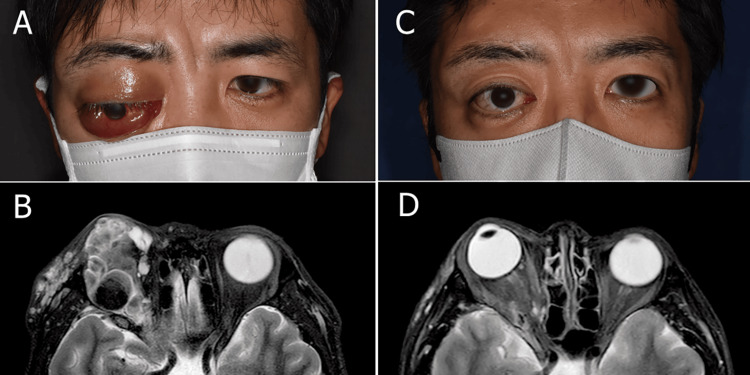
Case 2 (A) The patient before treatment showed distinct proptosis and chemosis. (B) The T2-weighted MRI image before treatment showed multiple small cystic lesions located above the superior rectus muscle, which were suspected to be intra-lesional hemorrhages, with a high signal around the lesions. Volumetry indicated that the lesion volume was 16.8 cm³. (C) The patient six months after starting sirolimus showed remarkable improvement in proptosis and chemosis. (D) The T2-weighted MRI conducted six months after starting sirolimus showed that the lesion volume was 3.8 cm³, and the reduction rate compared to before treatment was 82.1%. MRI: magnetic resonance imaging
